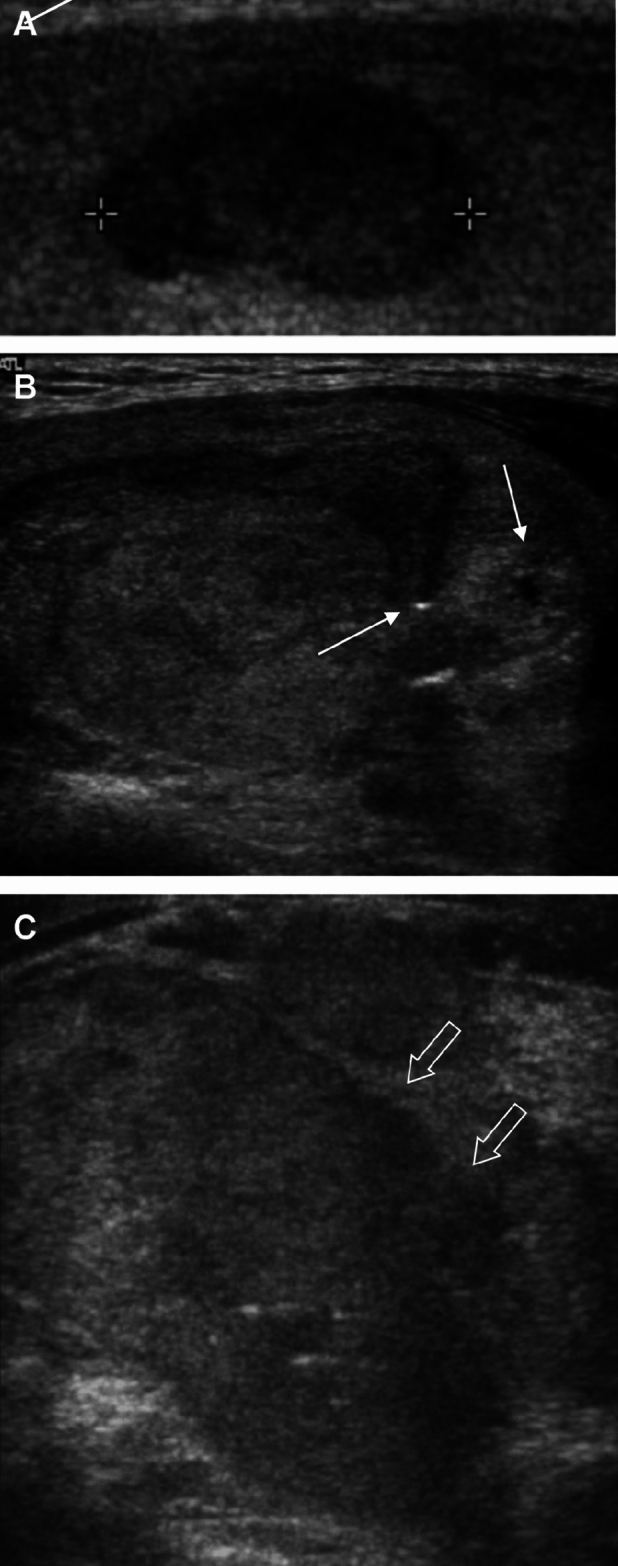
The TERT promoter mutated follicular thyroid carcinomas. Transverse ultrasound images show solid hypoechoic nodules with markedly decreased echogenicity (A), punctate echogenic foci (solid arrow) (B), or irregular margins (open arrow) (C). They were categorized as K-TIRADS/ACR-TIRADS category 4 (A and B), and K-TIRADS/ACR-TIRADS category 5 (C).
